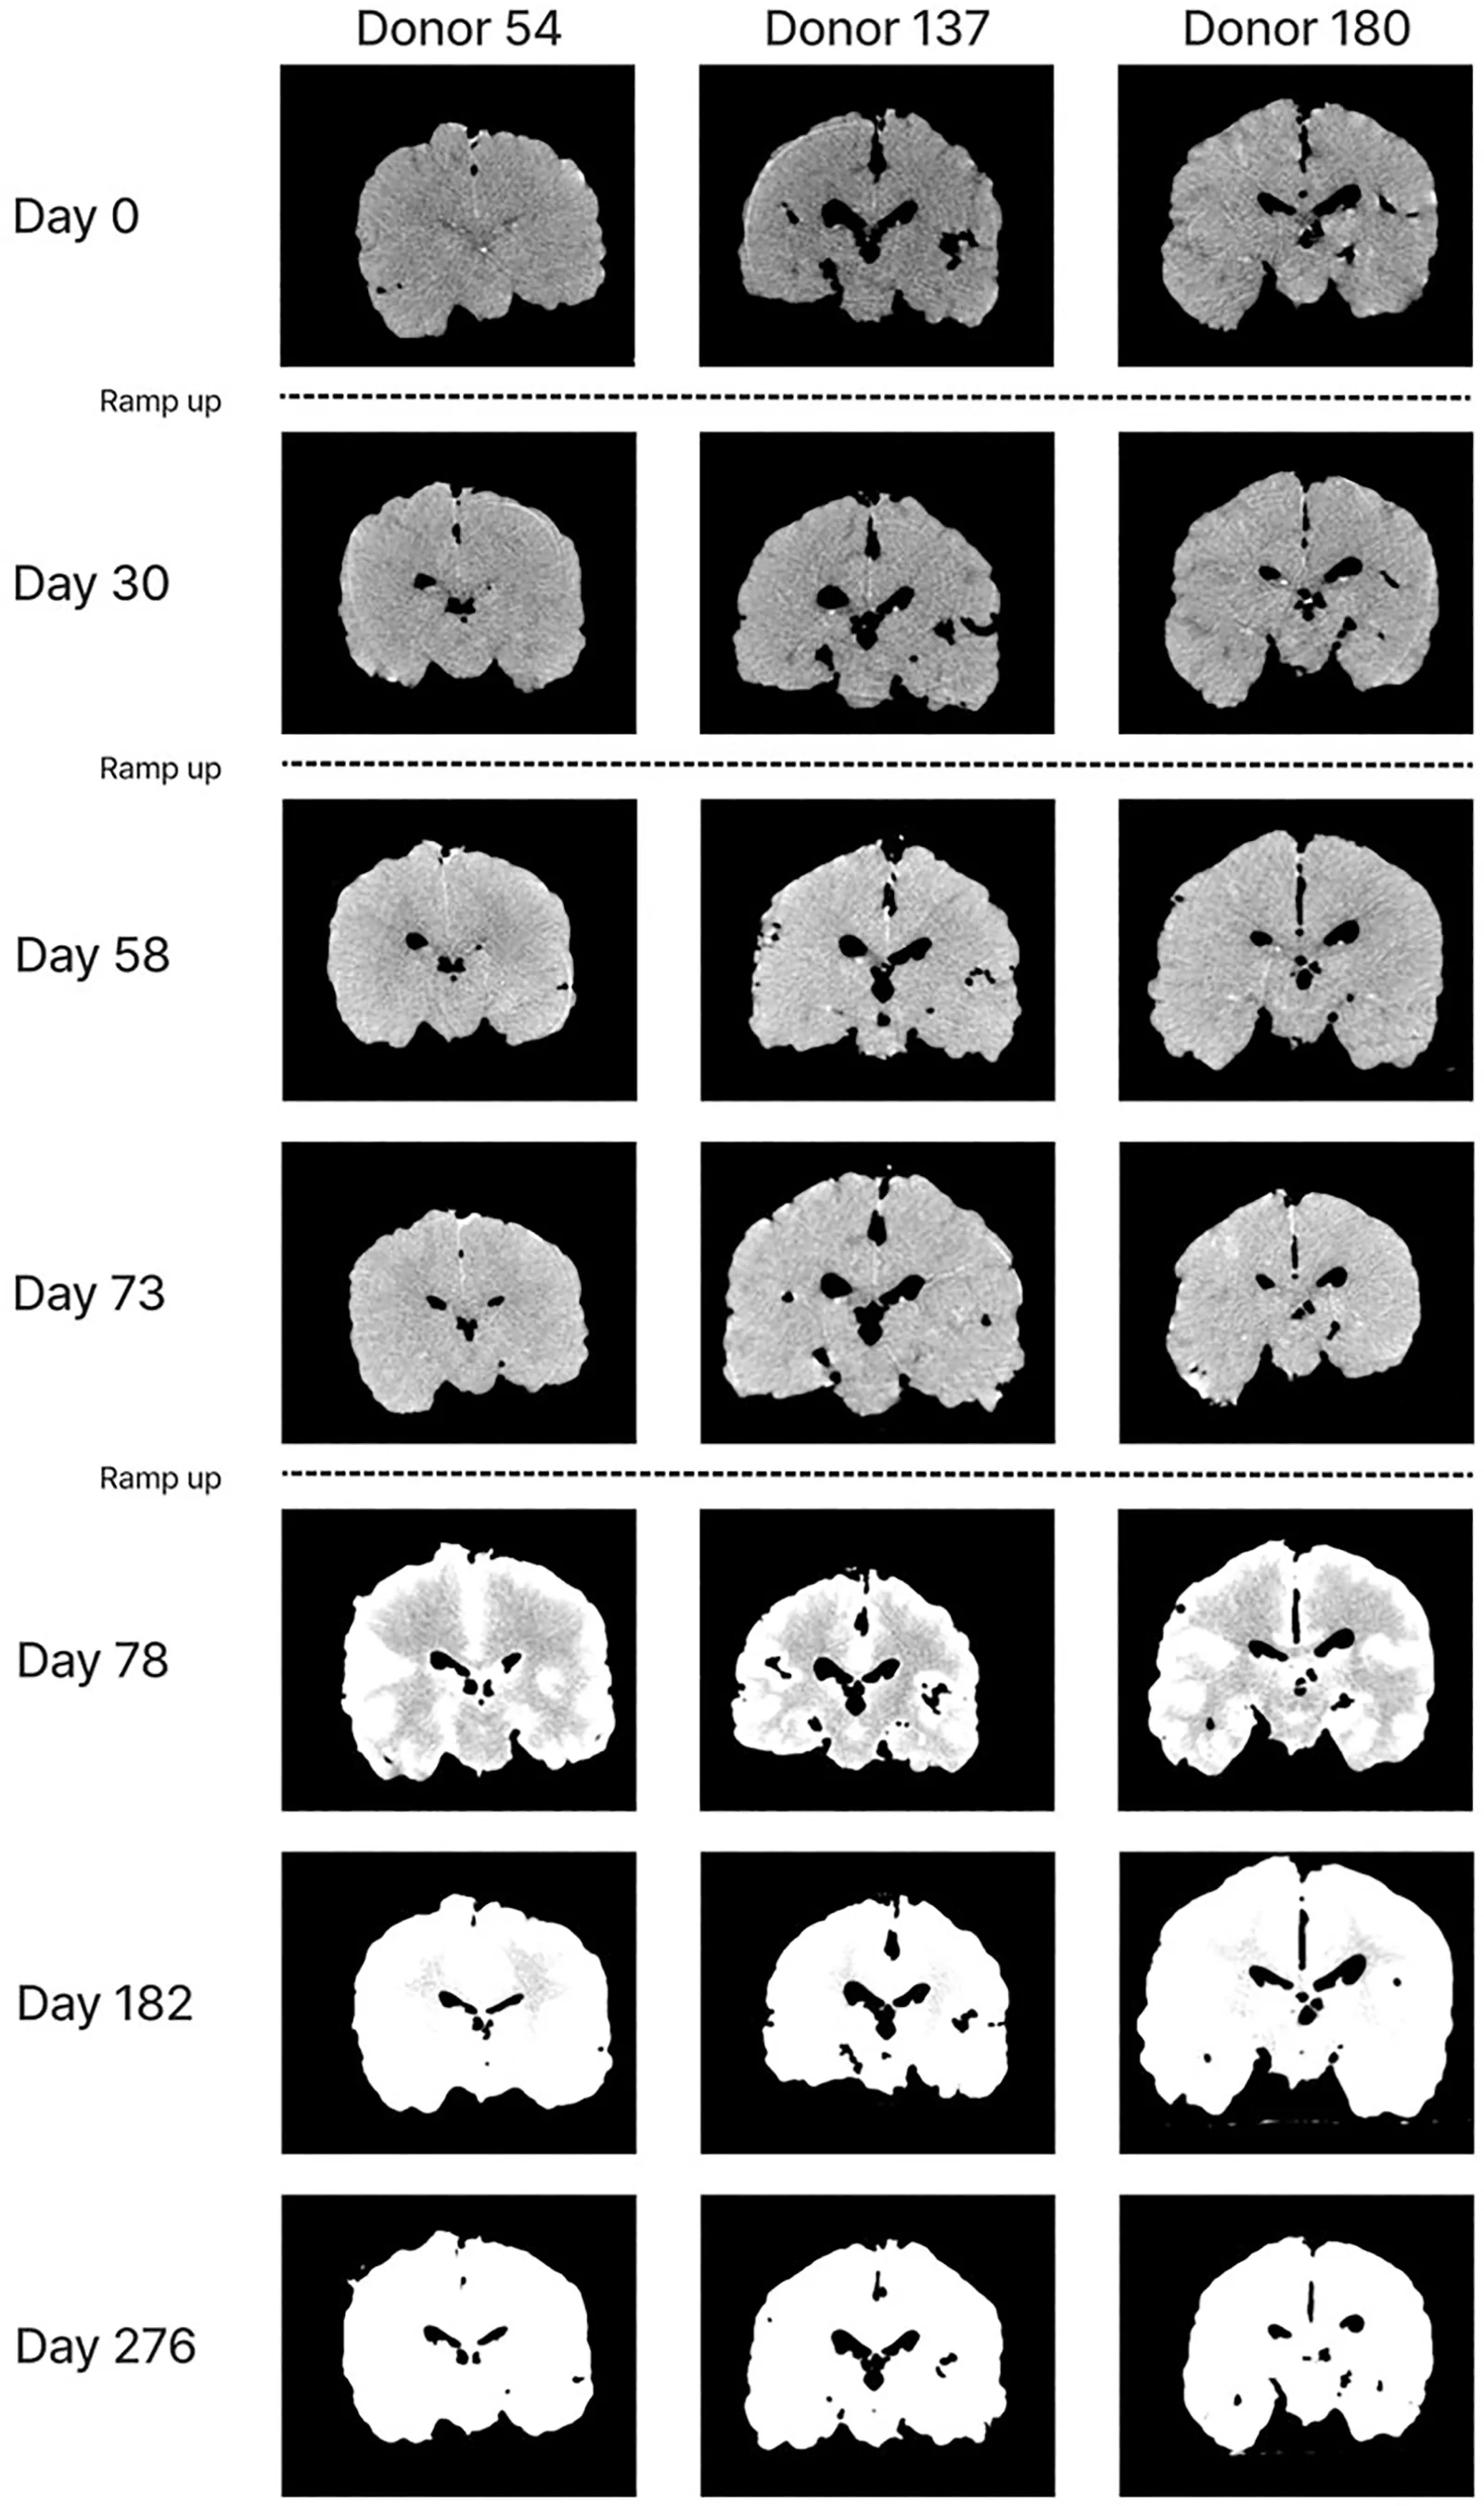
CT imaging of cryoprotectant penetration in whole human brains. Coronal CT sections from three donors (donor #54: 71-year-old female, postmortem interval (PMI) 117 hr; #137: 90 + -year-old male, PMI 47 hr; #180: 75-year-old male, PMI 46 hr) during stepwise cryoprotectant loading. Dashed lines indicate the concentration increases: 10% ethylene glycol (added on day 0), 30% ethylene glycol (added on day 30), and final solution containing 50% ethylene glycol, 30% sucrose, and 1% PVP-40 (added on day 73). Increasing brightness reflects rising Hounsfield Unit values as cryoprotectant diffuses inward. Note that grey matter equilibrates faster than white matter, with deep white matter regions showing delayed penetration. By day 276, the uniform signal intensity indicates equilibration throughout all of the brain.
